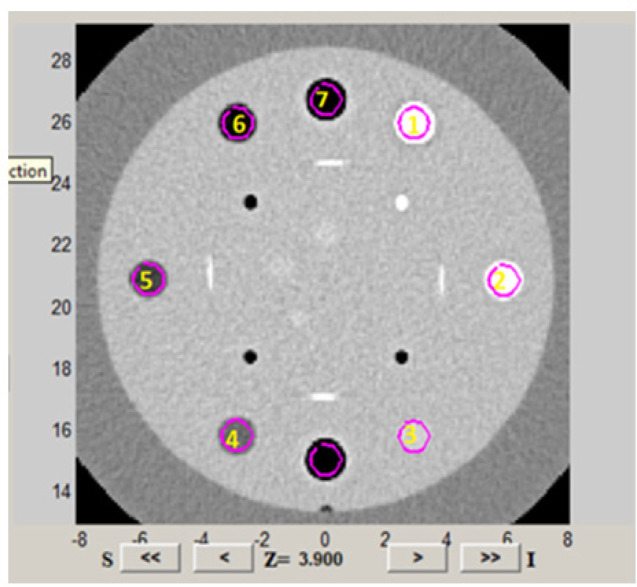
Axial Section Taken for the Analysis of CT Catphan® 504 Sensitometry Region Image with Segmented ROI Known Density Materials 1)Teflon , 2)Delrin , 3) Acrylic ,4) Polystyrene , 5) LDPE, 6) PMP and 7) Air
